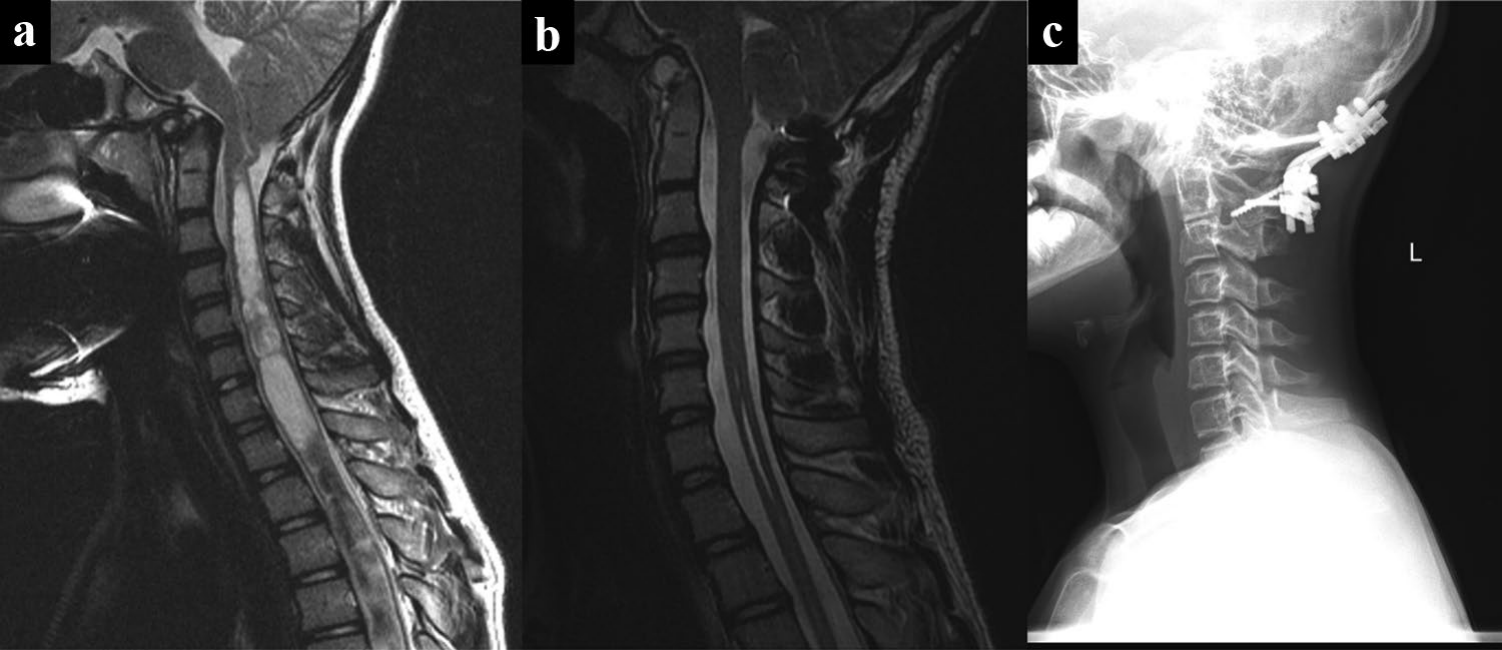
Effects of O-C2 fusion in patients with anterior pathology. a Preoperative T2-weighted mid-sagittal MRI demonstrating anterior pathology from basilar invagination and associated cervical cord syrinx. b Postoperative T2-weighted mid-sagittal MRI demonstrating reduction of anterior pathology after occipitocervical fusion to C2 utilizing the translaminar screw technique. c Lateral cervical spine XR demonstrating hardware with fusion
